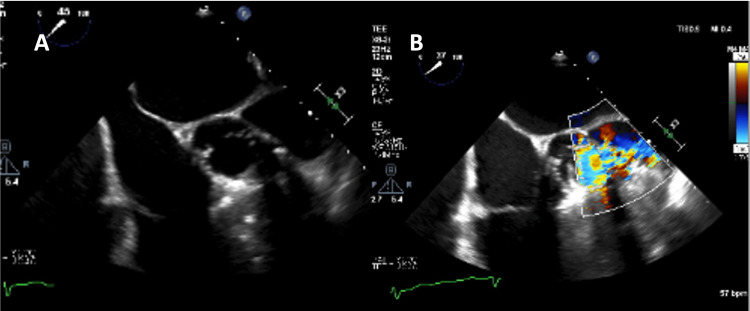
Transesophageal echocardiography demonstrating left coronary sinus of Valsalva and aortic ring rupture with pseudoaneurysm (A) and systolic flow by color flow Doppler (B).
